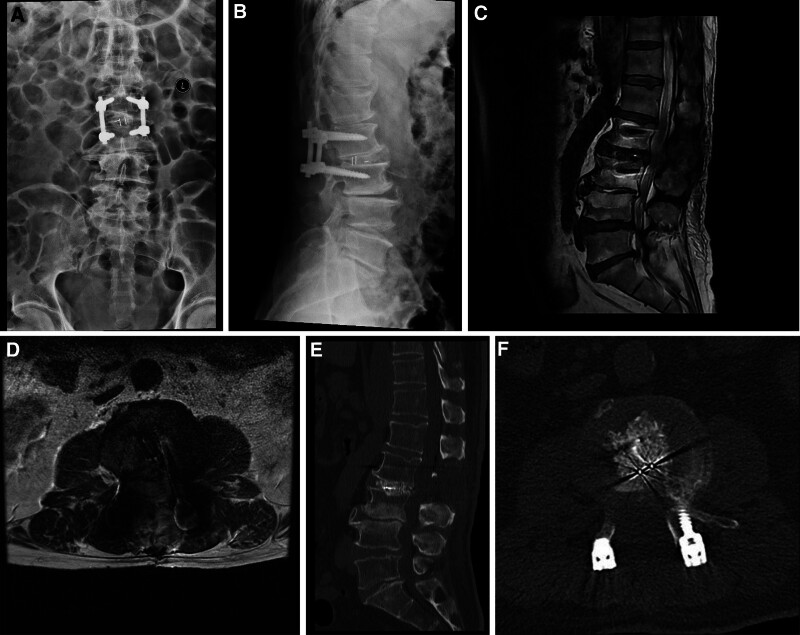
(A, B) Frontal and lateral DR of the lumbar spine, (C) T2W sagittal image, (D) T2W axial image, and (E) sagittal and (F) axial CT images demonstrating that there was no longer a buildup of gas in the spinal canal, and that there was adequate decompression of the spinal canal. CT = computed tomography, DR = digital radiography.
